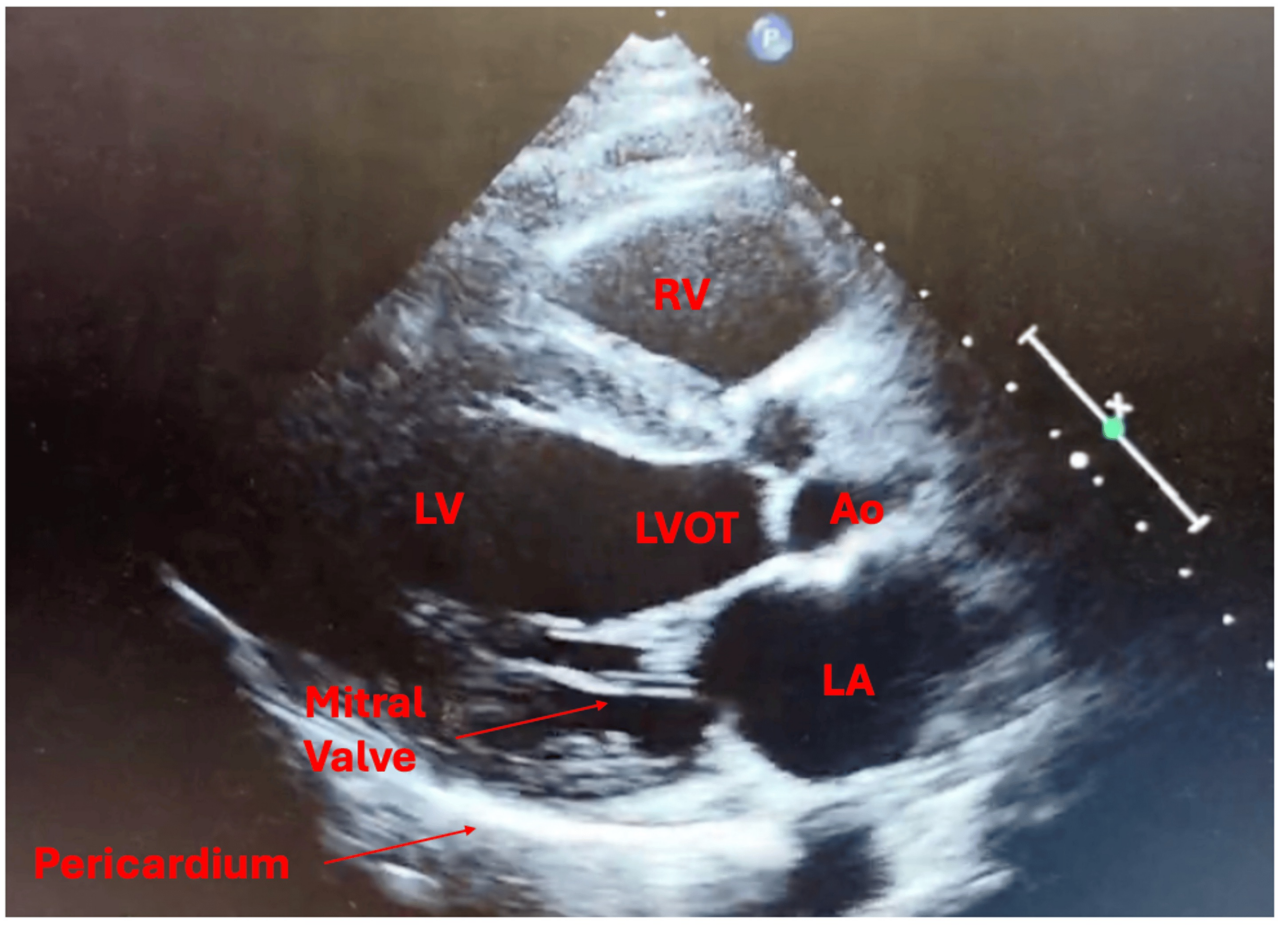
Parasternal long-axis transthoracic echocardiogram. The RV is not dilated, consistent with no RV strain. The pericardium is visualized without evidence of pericardial effusion. RV: right ventricle; LV: left ventricle; LA: left atrial; LVOT: left ventricular outflow tract
